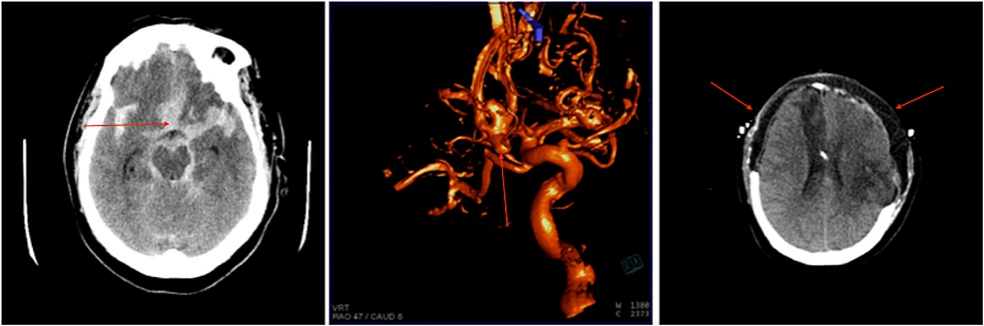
Imaging related to the clinical course of patient 4. (A) Head CT obtained on presentation demonstrated SAH secondary to a presumed anterior communicating artery aneurysm. (B) Angiogram obtained after presentation demonstrating an anterior communicating artery aneurysm and left middle cerebral artery aneurysm. (C) Post-operative head CT after bilateral DCHC for refractory elevated ICP. SAH, subarachnoid hemorrhage; DCHC, decompressive hemicraniectomy; ICP, intracranial pressure
